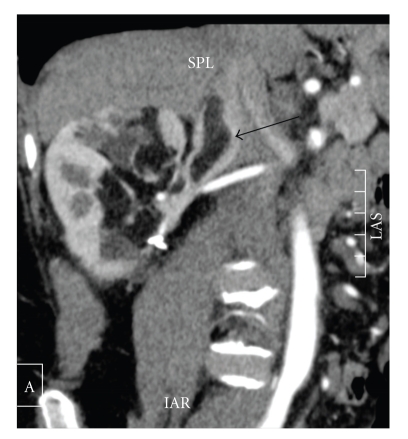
Computed tomography scan demonstrating right, fat containing, renal mass with renal vein and vena cava involvement (arrow).
